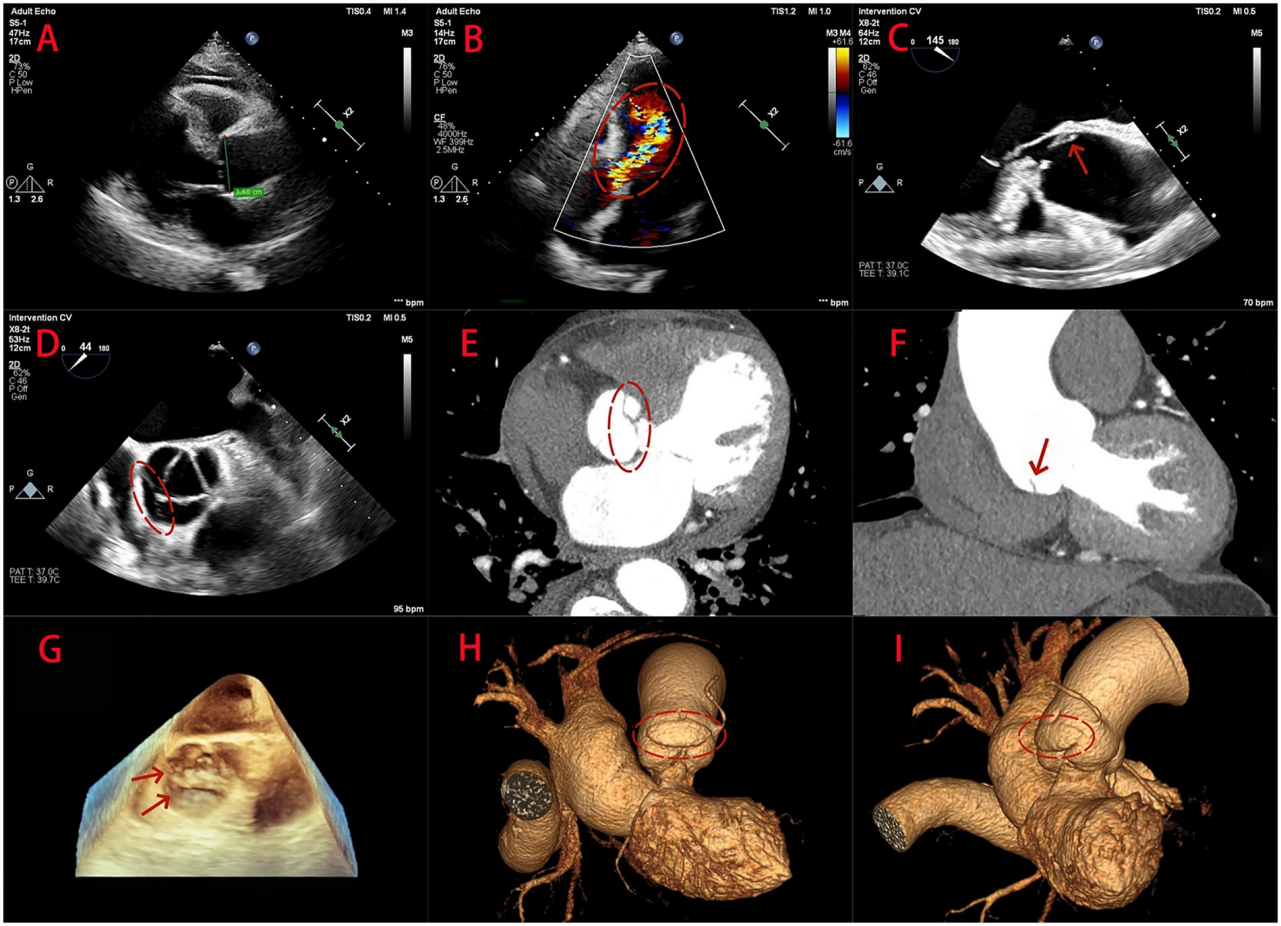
Echocardiographic and coronary computed tomography angiography (CTA) findings in a 68-year-old man with localized aortic root dissection. (A) Emergency transthoracic echocardiography (TTE) on admission shows no aortic root dilatation, with the sinuses of Valsalva measuring 36 mm. (B) Doppler demonstrates severe aortic regurgitation (red circle). (C,D,G) Preoperative transesophageal echocardiography (TEE) in two- and three-dimensional views demonstrates a distinct, obliquely oriented membranous structure confined to the aortic sinus, involving the right and non-coronary sinuses (red arrows/circle). (E,F) Retrospective review of the preoperative coronary CTA identifies a localized, obliquely oriented intimal flap within the aortic sinuses on coronal and axial images (red arrow). (H,I) Three-dimensional reconstructions demonstrate mild focal bulging of the involved sinus walls in the dissected segment (red circle).
